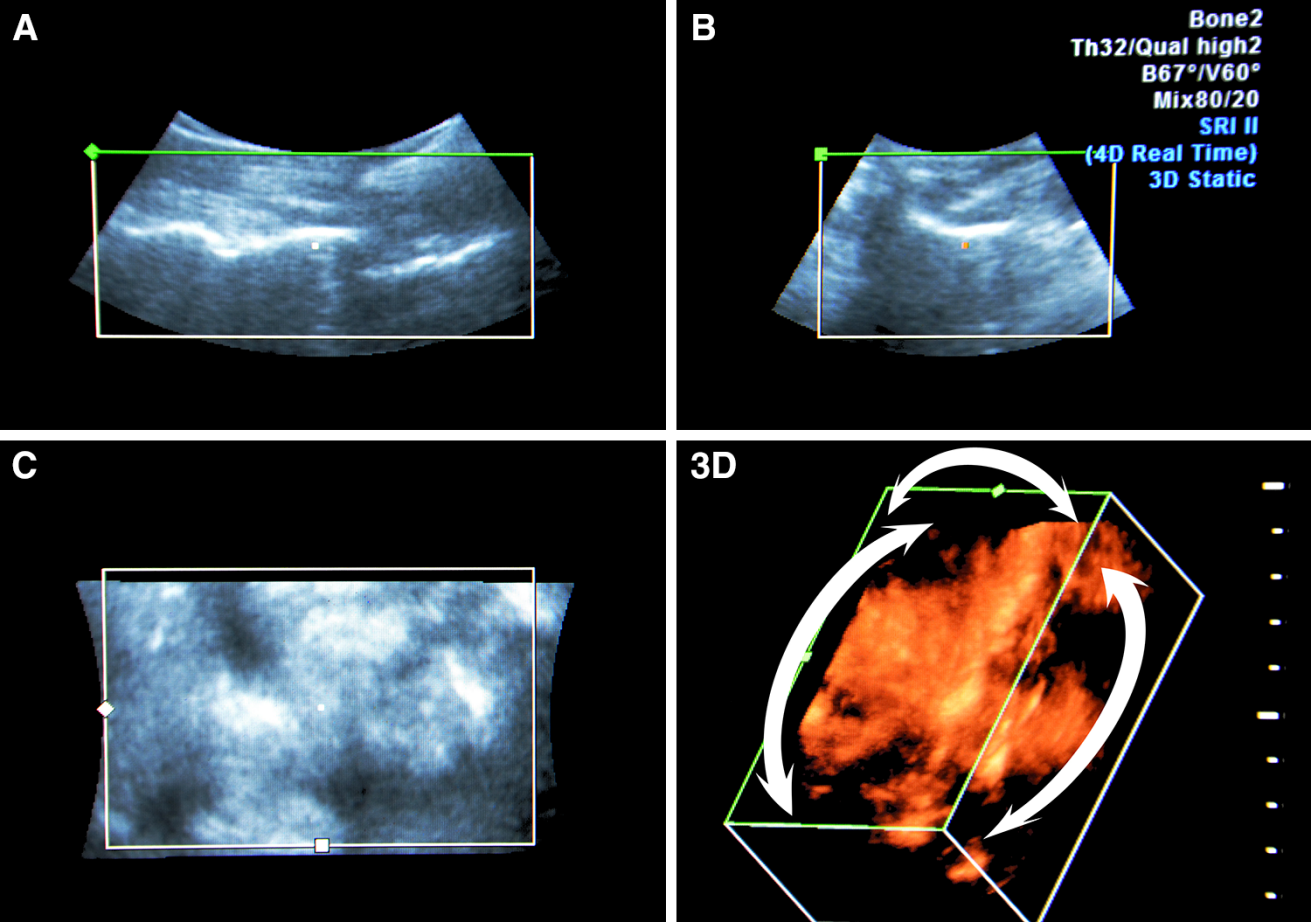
Paramedian sagittal sonogram of the lumbar spine. *A* is the Longitudinal paramedian image, *B* is the axial image, *C* is the coronal image, and *3D* is the three-dimensional reconstruction with frame reconstruction (set by the operator). Note that the *3D* image can rotate at any angle (*white arrows*), and the location and image slices *A*, *B*, and *C* remain unchanged. Additionally, the operator can change the viewing scale of the image to see the details and improvements or, in contrast, zoom out to encompass the whole panorama
